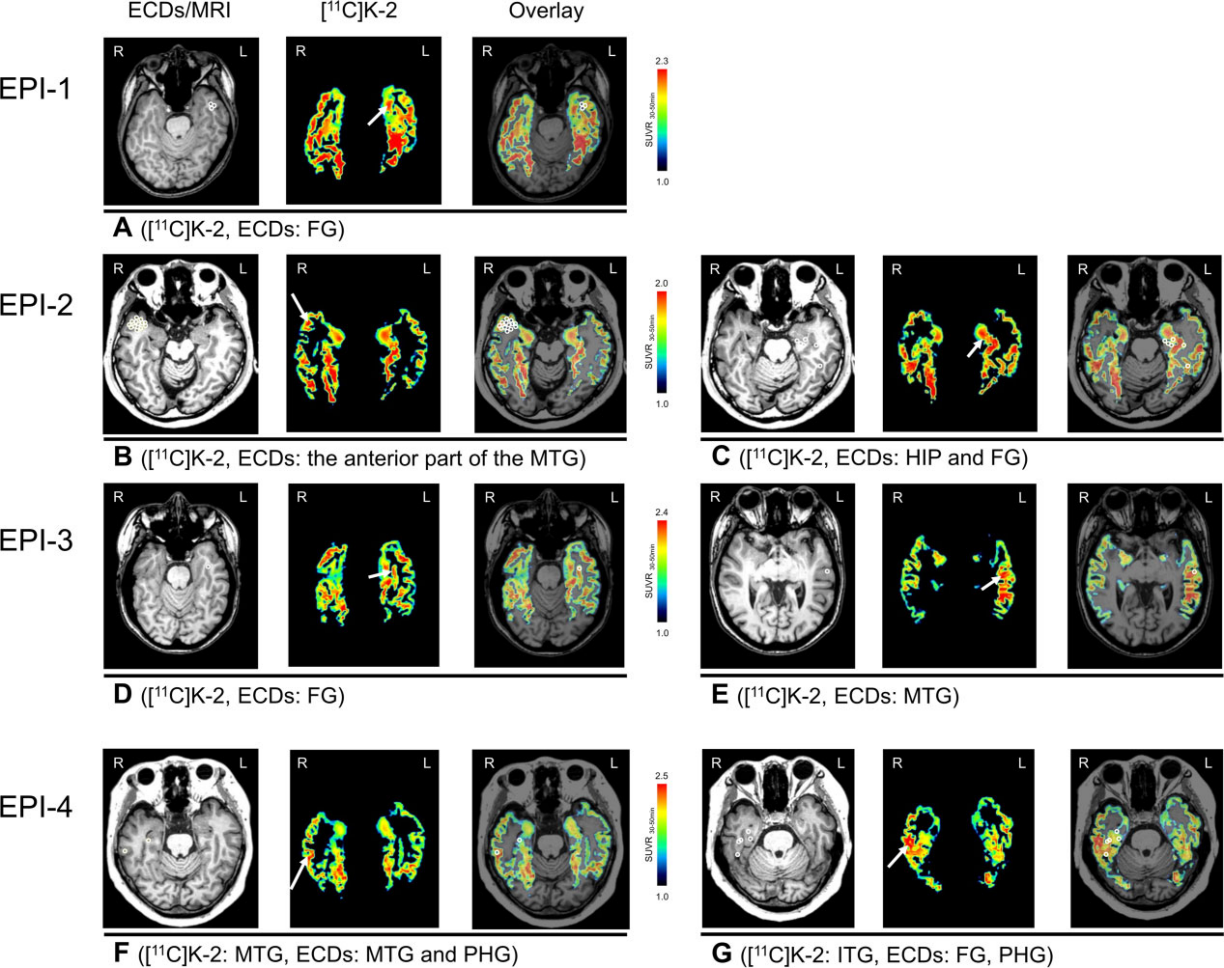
Increased [^11^C]K-2 signals colocalize with ECDs in SUVR_30–50 min_ images of temporal lobes in patients with MTLE. Representative [^11^C]K-2 SUVR_30–50 min_ images are shown restricted to temporal lobes where ECDs are estimated. Each panel shows ECDs on MRI (left), [^11^C]K-2 (middle) and overlay (right). White arrows indicate the area showing elevated [^11^C]K-2 uptakes and white circles indicate estimated ECDs. (A) EPI-1 showed elevated [^11^C]K-2 uptake and estimated ECDs in the left FG. EPI-2 showed elevated [^11^C]K-2 uptake and estimated ECDs in the anterior part of the right MTG (B) and, further, elevated [^11^C]K-2 uptake and estimated ECDs were identified in the left HIP and the anterior part of FG. One ECD (posterior white circle) was estimated in the posterior part of FG (C). EPI-3 showed elevated [^11^C]K-2 uptake (white arrow) and estimated ECD (white circle) in the left FG (D) and, further, elevated [^11^C]K-2 uptake and estimated ECDs were identified in left MTG (E). EPI-4 showed elevated [^11^C]K-2 uptake (white arrow) and estimated ECD (outer white circle) in MTG and the other ECD was estimated in the parahippocampal gyrus (PHG, inner white circle) (F). Furthermore, elevated [^11^C]K-2 uptake was identified in the right ITG and estimated ECDs were identified in FG, adjacent closely to ITG, PHG (G).
